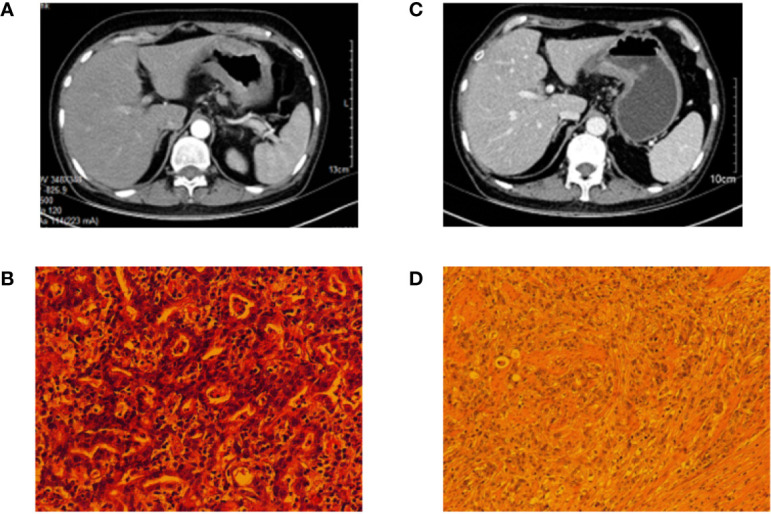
Pathology and imaging data of patient 1. (A) The CT of the patient at the first examination; (B) The gastroscopic pathology that suggested adenocarcinoma of the gastric body. (C) The CT of the patient after four cycles of combined chemotherapy. (D) Postoperative pathology of the patient.
